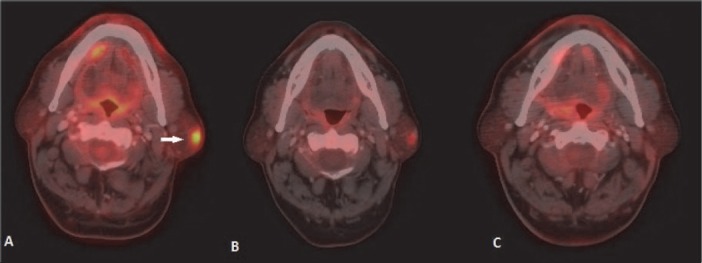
Lymphoma of left parotid gland: Same patient as in figure 4. (A) Relapsed follicular lymphoma shows FDG avid nodule in superficial lobe of the left parotid gland. Patient also had nodal disease including cervical nodes. (B) Post 3 cycles Bendamustine shows complete metabolic response. (C) Follow up scan after 6 cycles of chemotherapy shows no residual lesion in left parotid gland
